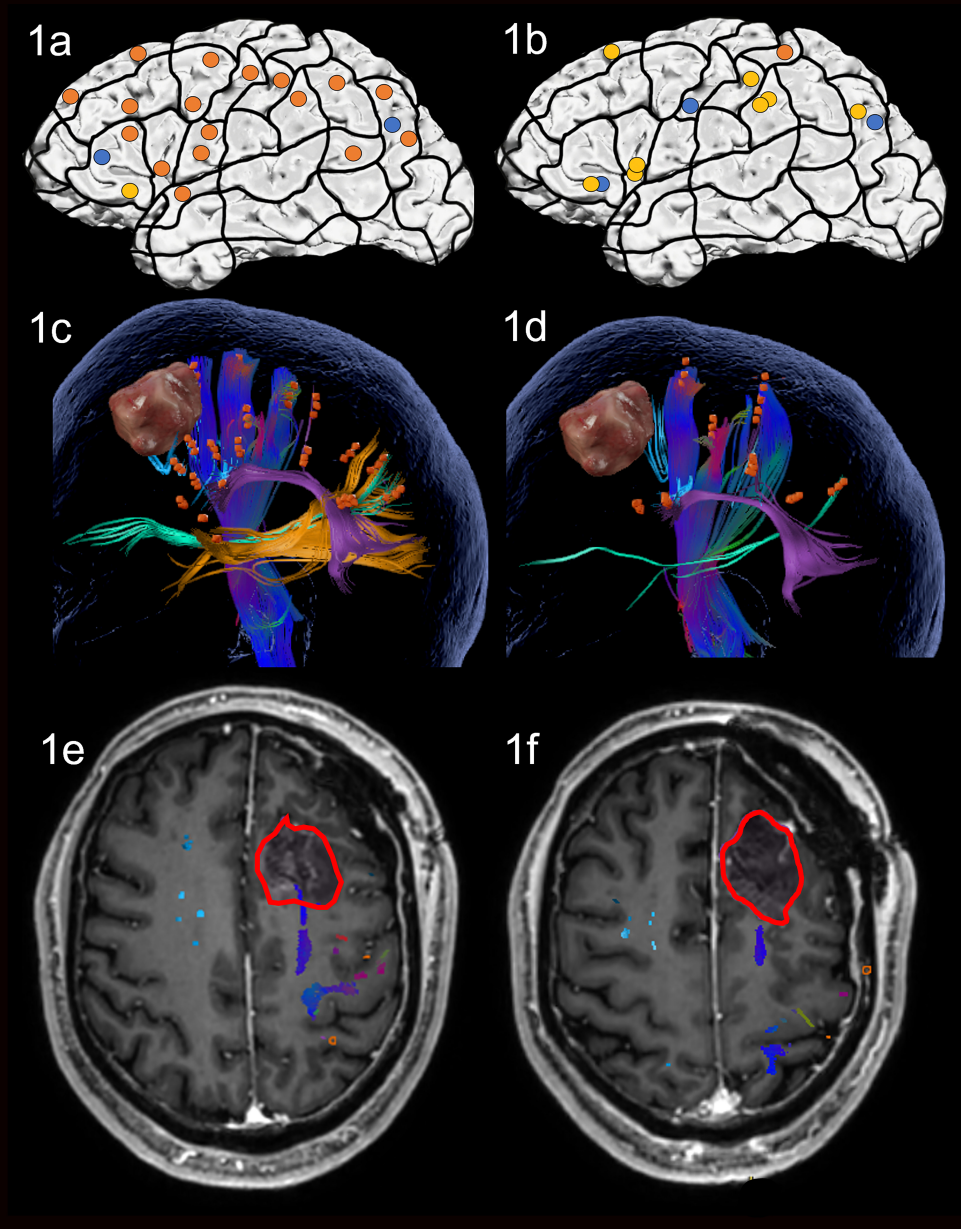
Case 1. Left panels show ON results, right panels AN results. Panels (A, B) nTMS stimulation sites that induced errors; no response and hesitations in orange, semantic errors in yellow and performance errors in blue. Panels (C, D) Fiber tracking results including the lesion, nTMS cortical endpoints in orange and color-coded tracts with CNT in direction-coded, here dark blue, AF in purple, IFOF in turquoise, ILF in yellow, and CF in light blue. Panels (E, F) Overlap of tracking and postoperative T1-weighted imaging after elastic fusion with the resection cavity marked in red.
